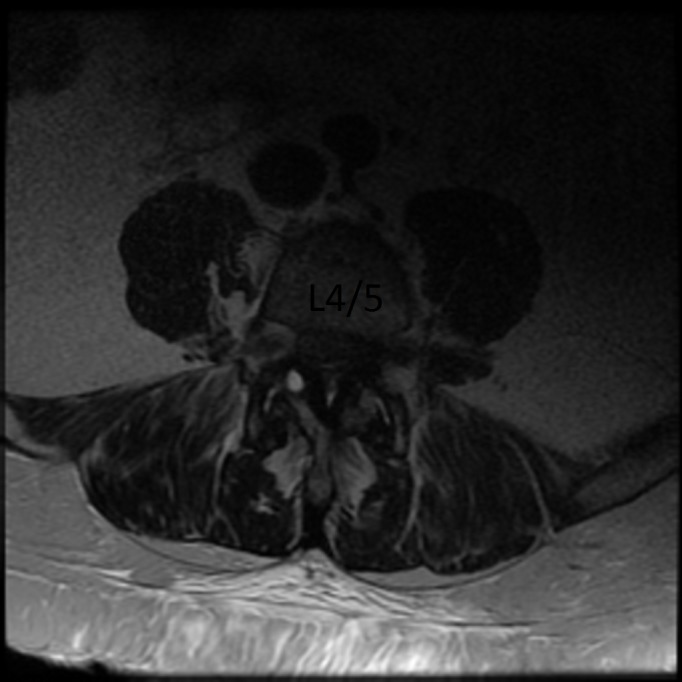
Pre-op Axial Section L4-5: MRI of Lumbar Spine
